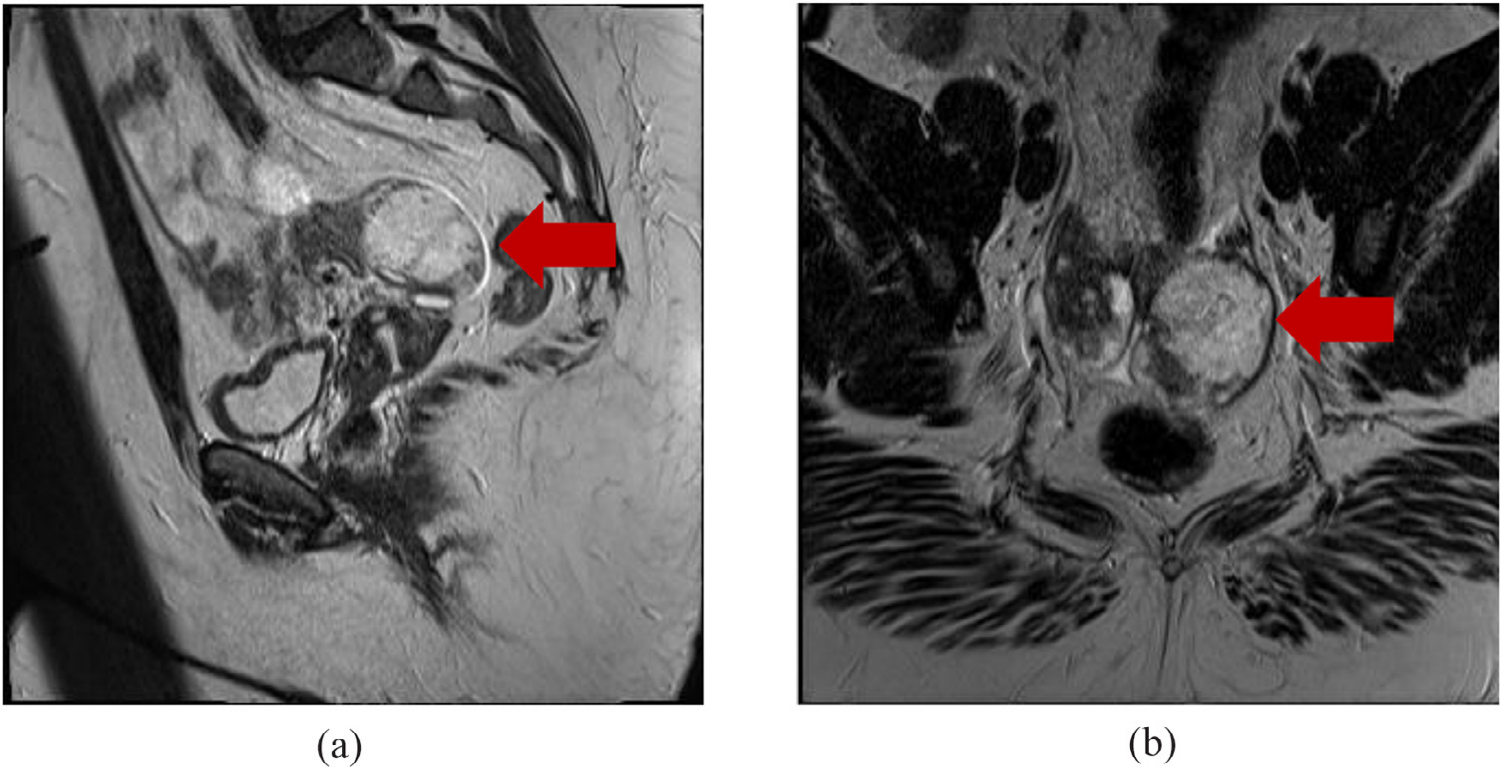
(A) MRI of the pelvis. Sagittal T2-weighted image showing a hyperintense left ovarian mass. (B) MRI of the pelvis. Axial T2-weighted image showing a hyperintense left ovarian mass.
